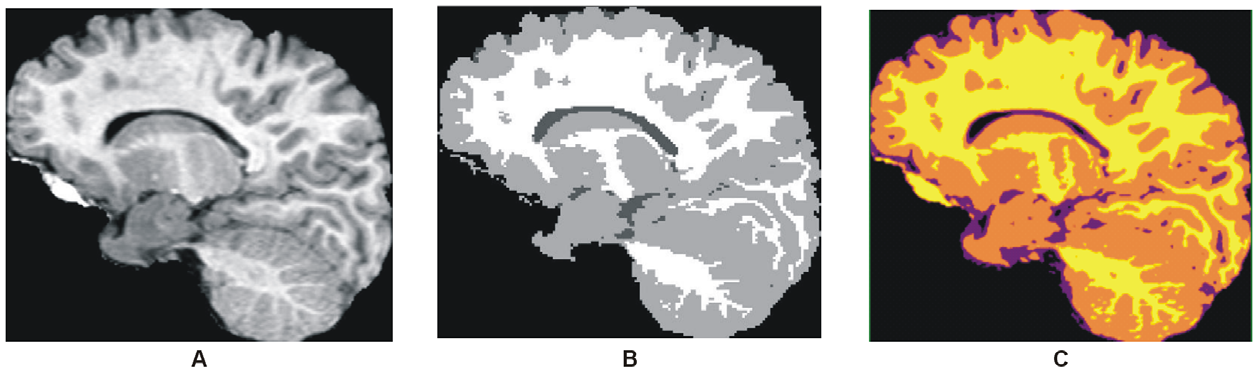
Comparison among the results obtained with our proposed segmentation method and EM-based segmentation. (A) T1 brain MR image of 2 year old child. (B) Segmented image with EM segmentation method. (C) Segmentation by proposed method.
